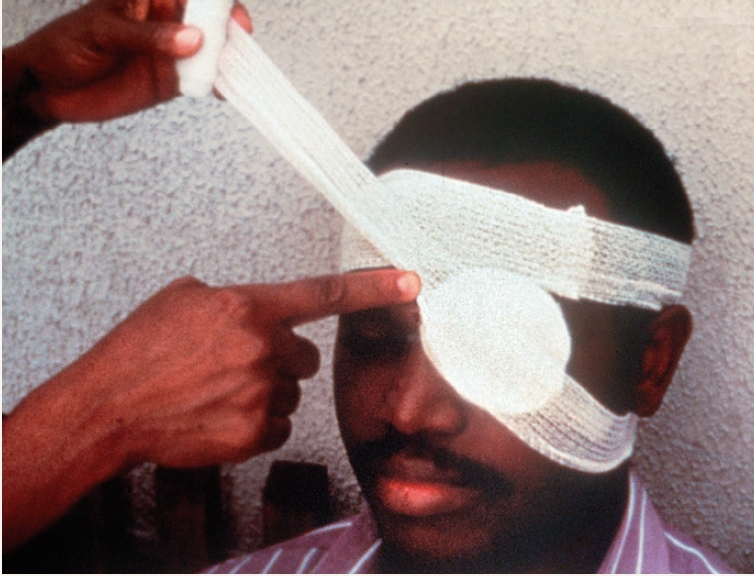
Making an eye pad and eye shieldYou will needcotton wooltwo pieces of gauzescissorsadhesive tapethin cardboard or old X-ray filmcircular object - about 8 centimetres in diameterpencil
